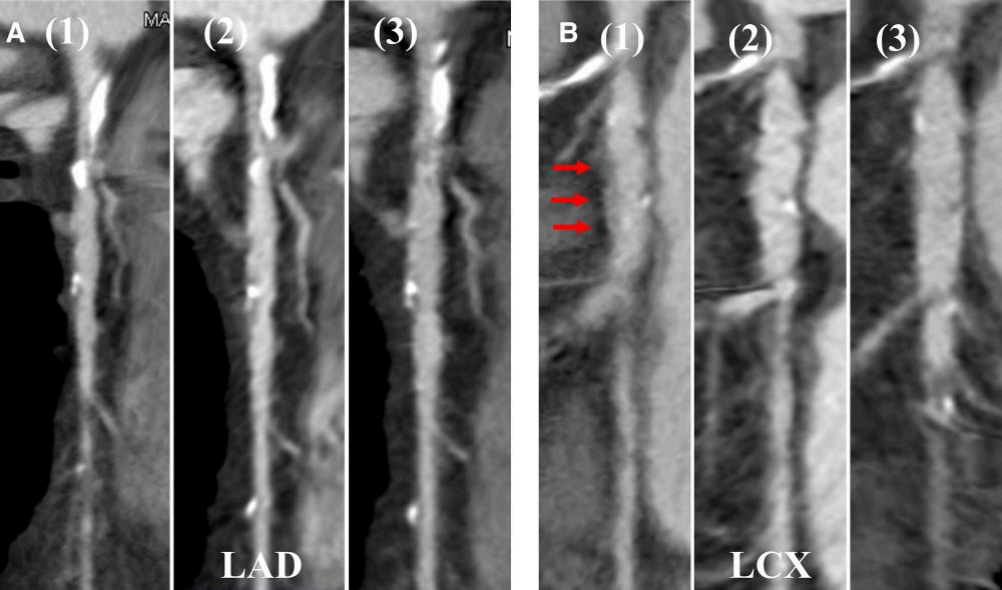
Serial changes in coronary computed tomography angiography of the left coronary artery. Serial changes of coronary computed tomography angiography findings of the left coronary artery are shown. (*A*) The left anterior descending artery. (*A*1) Pre-intervention. (*A*2) Fourteen months later. (*A*3) Twenty-six months later. Note no change in the size of the aneurysm. (*B*) Left circumflex artery. The arrows in (*B*1) show diffuse perivascular soft tissue thickening, which gradually disappeared after 14 (*B*2) and 26 (*B*3) months.
